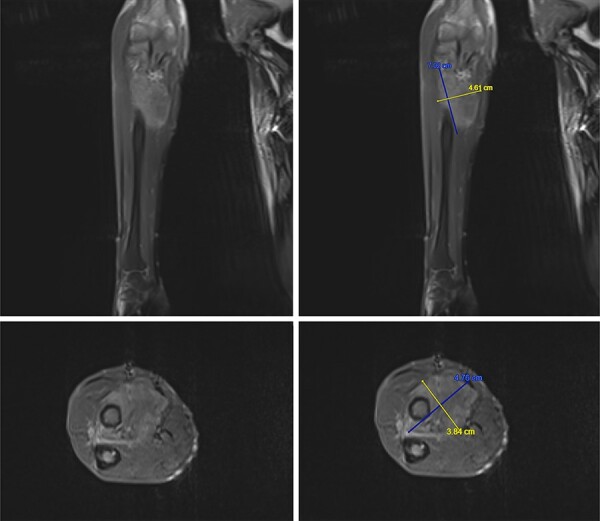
MRI T1 Turbo-spin-echo (TSE) of the right forearm showing the intermuscular enhancing lesion at the volar aspect of the proximal radius distal to the radial tuberosity interposed deep to the flexor and extensor muscles. The lesion measures approximately 7 cm × 4.6 cm × 3.84 cm.
